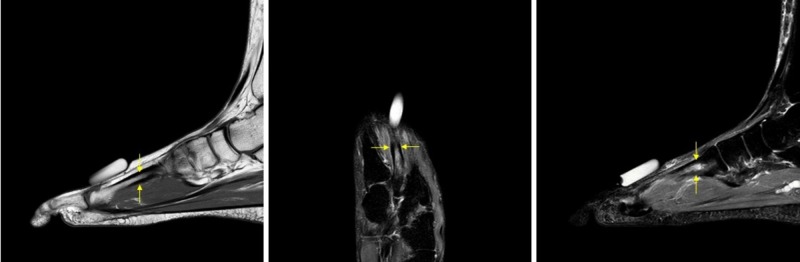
Magnetic resonance imaging (MRI) showing stress reaction of the second metatarsal prior to cement injection. From left to right: sagittal T1; Coronal STIR; sagittal STIR.
